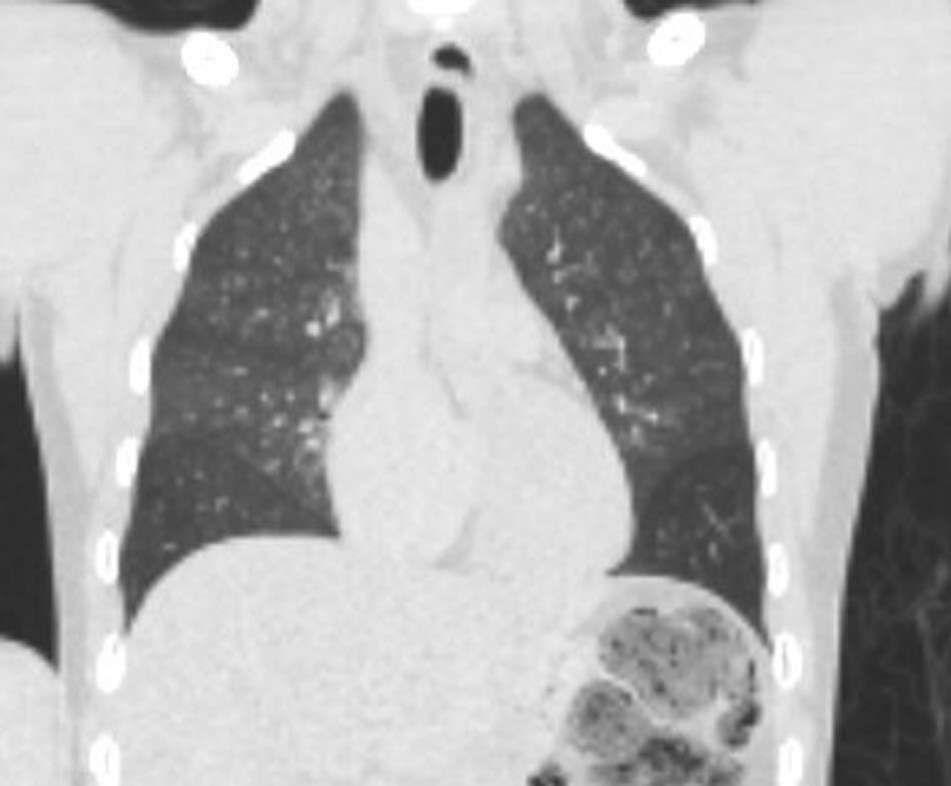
Postmortem CT (PMCT) coronal chest: one 30-year-old male with complaints of dizziness and nausea. He was found unresponsive in bed. Postmortem SARS-CoV-2 was positive, but the lungs are clear of consolidation or pulmonary findings of acute respiratory distress syndrome (ARDS). The primary cause of death on the official record was drug overdose due to fentanyl toxicity.
